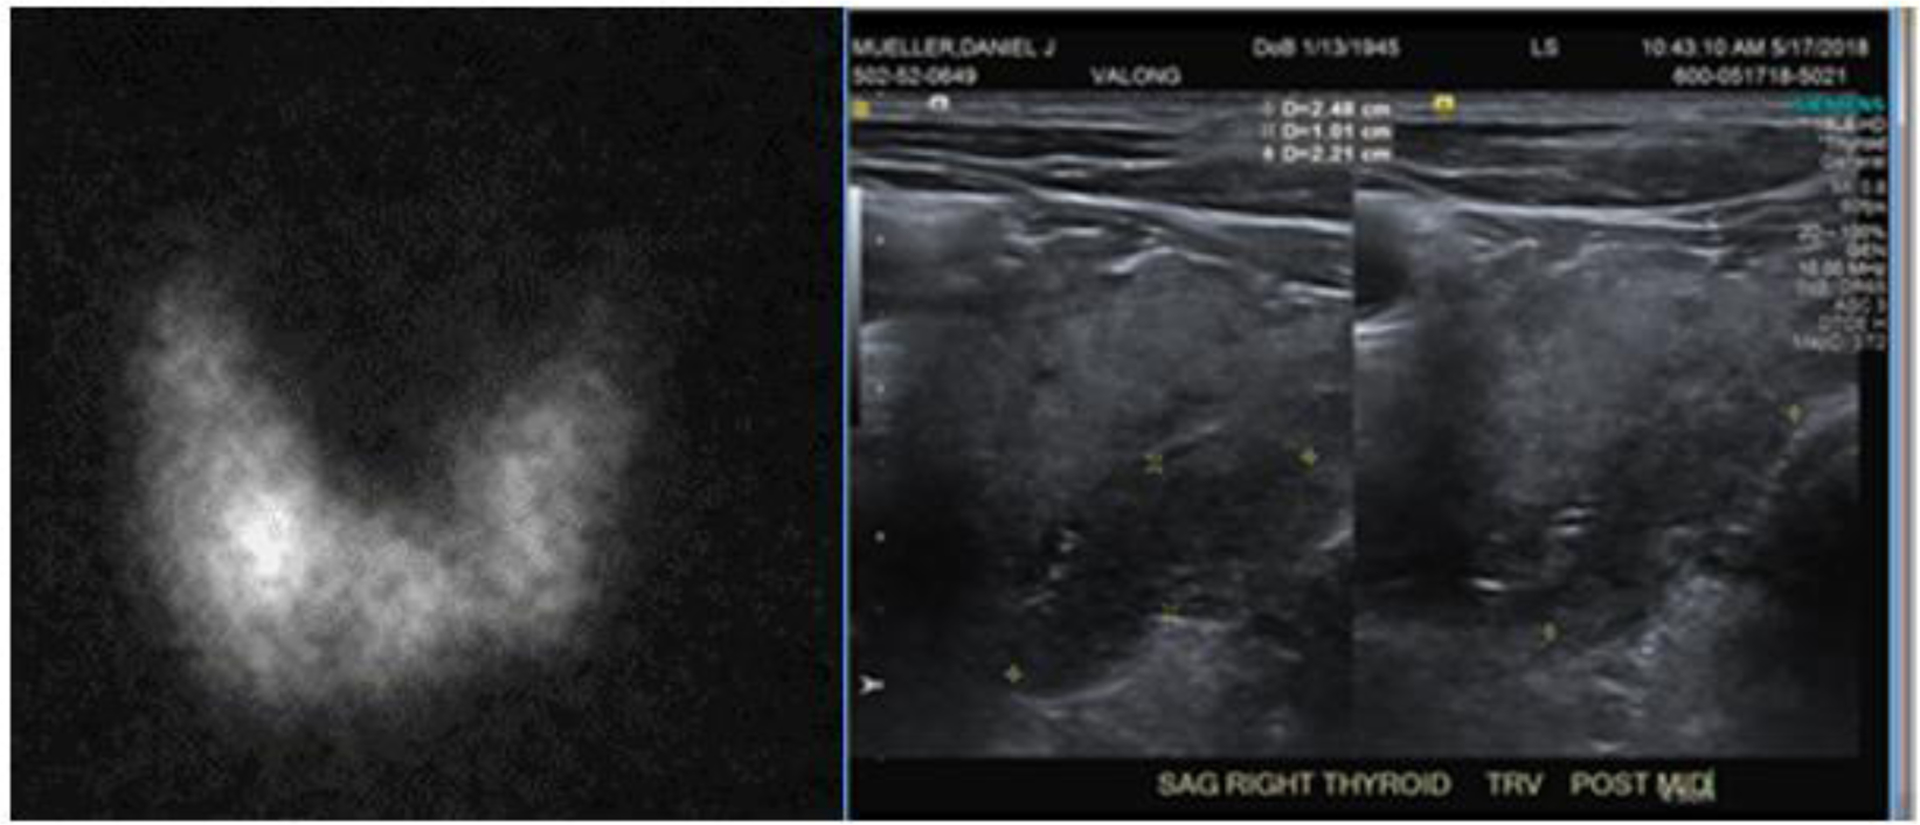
Patient A: (Left) I-123 thyroid scan on 6/27/2018 showed diffusely increased uptake bilaterally (57.5% at 24 h, normal range: 8–35%) and a hot thyroid nodule in the right lower lobe. (Right) Thyroid ultrasound examination on 5/17/2018. The image is the sagittal (long) and trans-axial images There is a 1.8 × 1.3 × 2.1 cm predominantly solid nodule in the medial right lower lobe.
